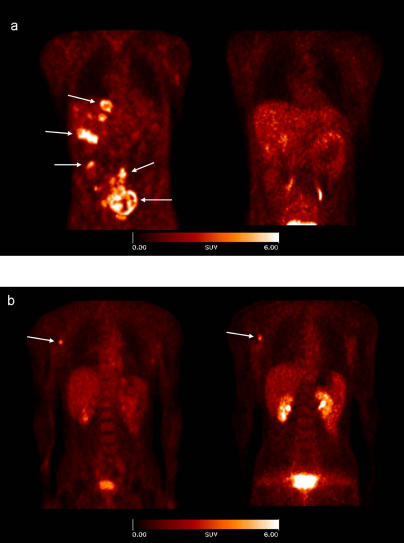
Comparison of [^18^F]FDG and [^18^F]Galacto-RGD Scans Coronal image sections, acquired 60 min p. i. (A) Patient with malignant melanoma stage IV and multiple metastases in liver, skin, and lower abdomen (arrows): marked uptake of [^18^F]FDG in the lesions (left), but no uptake of [^18^F]Galacto-RGD (right). (B) Patient with malignant melanoma stage IIIb and a solitary lymph node metastasis in the right axilla (arrow): intense uptake of both [^18^F]FDG (left) and [^18^F]Galacto-RGD (right).
